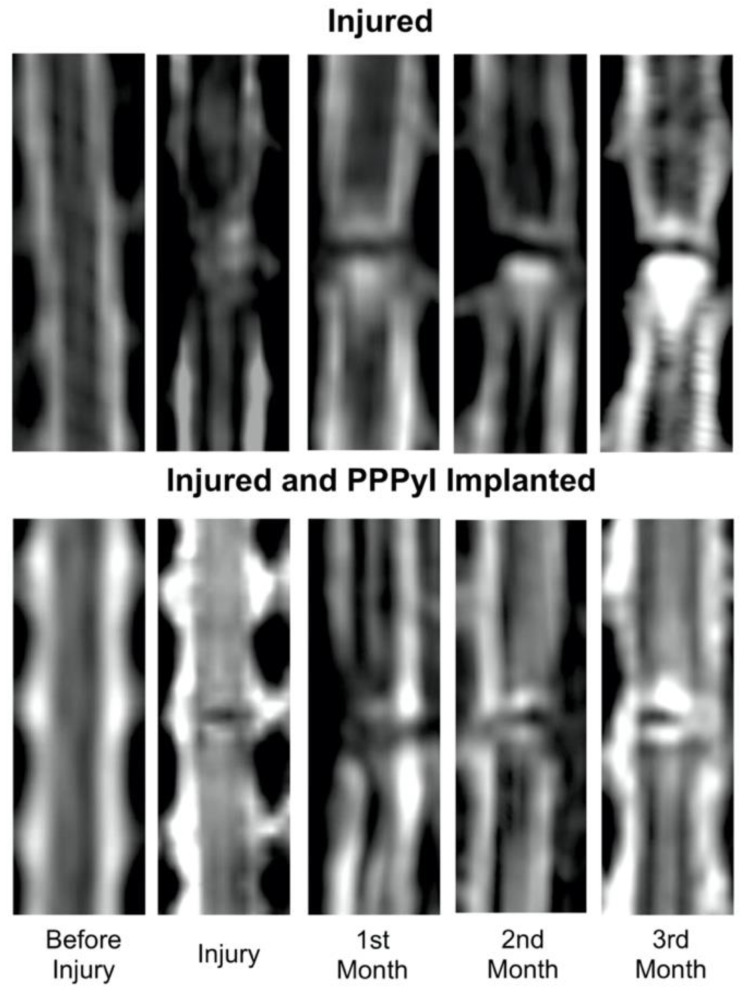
T2-weighted magnetic resonance imaging study of Rhesus injured and injured + implanted. T2W sagittal reconstruction images show transection site from before injured up to 3 months after injury.
